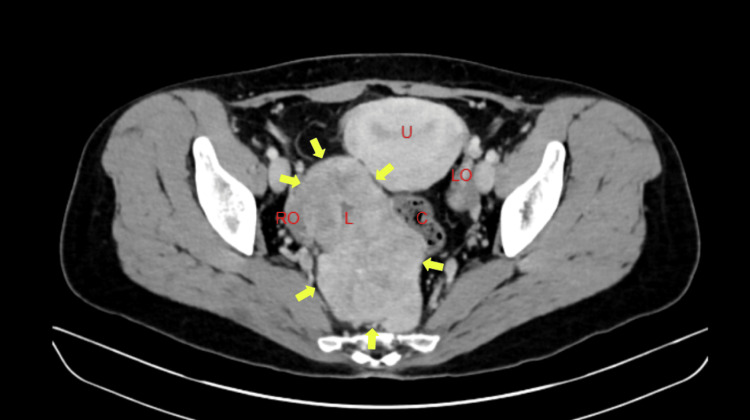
A CT Abdomen/Pelvis showing leiomyoma in transverse view. Yellow arrows showing leiomyoma border. U - Uterus, RO - Right Ovary, LO - Left Ovary, L - Leiomyoma, C - Colon.
